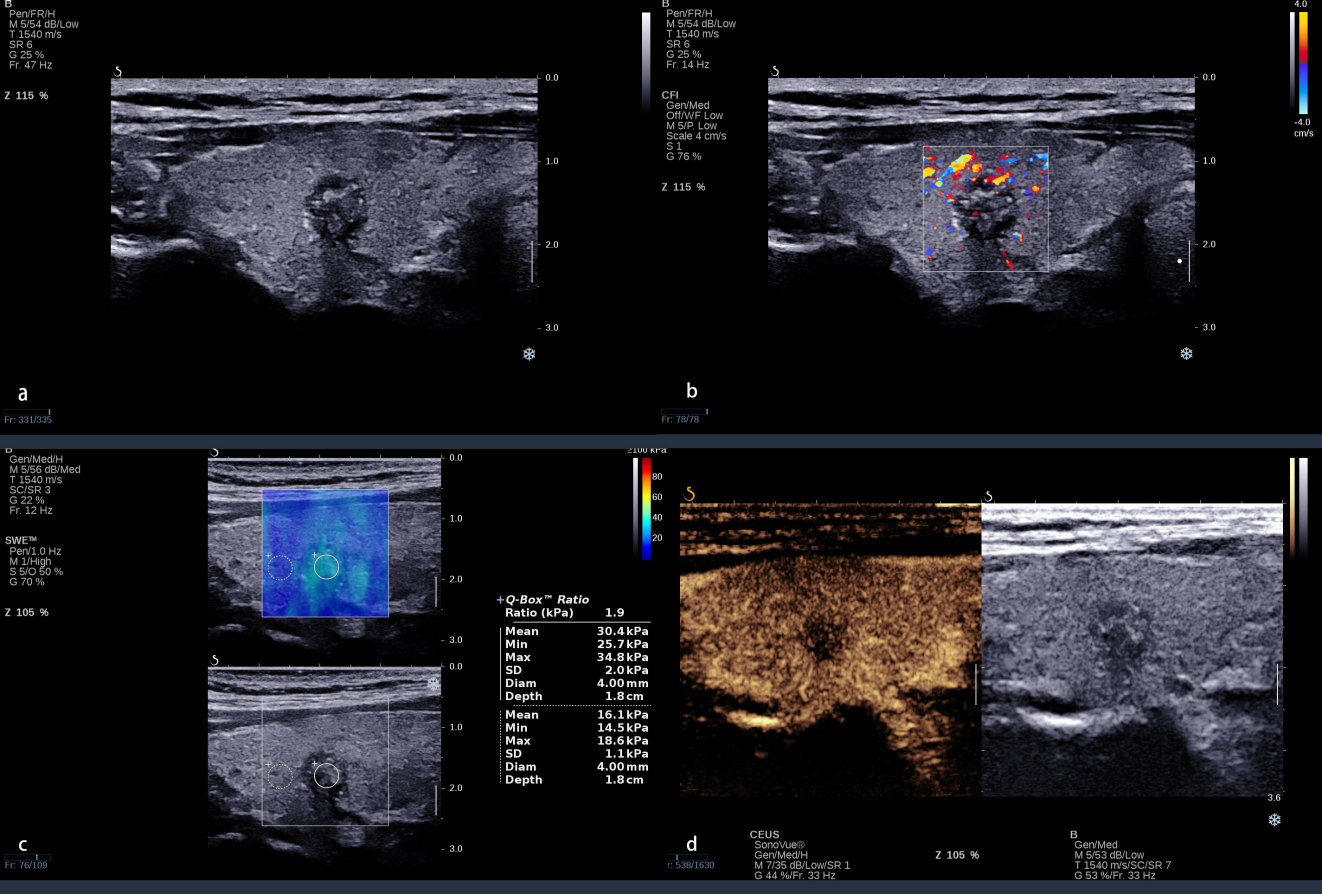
A 38 year-old-woman with a nodule in the right lobe of the thyroid. (A) Conventional ultrasound revealed an 8 × 9-mm solid hypo-echoic nodule with an irregular margin, shape that was taller-than-wide, and with punctate echogenic foci; this was categorized ACR TI-RADS 5. (B) The nodule presented a spot blood flow signal. (C) Quantitative shear wave elastography (SWE) revealed 25.7 kPa for Emin, 30.4 kPa for Emean, 34.8 kPa for Emax, and 1.9 for Eratio. (D) Qualitative contrast-enhanced ultrasound (CEUS) of the nodule revealed heterogenous, hypo-enhancement. Even the Emax was <46.8 kPa; the CEUS presented malignant enhancement patterns. The combination of CEUS and SWE recognized this nodule as malignant. The surgical pathology was papillary thyroid carcinoma with Hashimoto’s thyroiditis.
